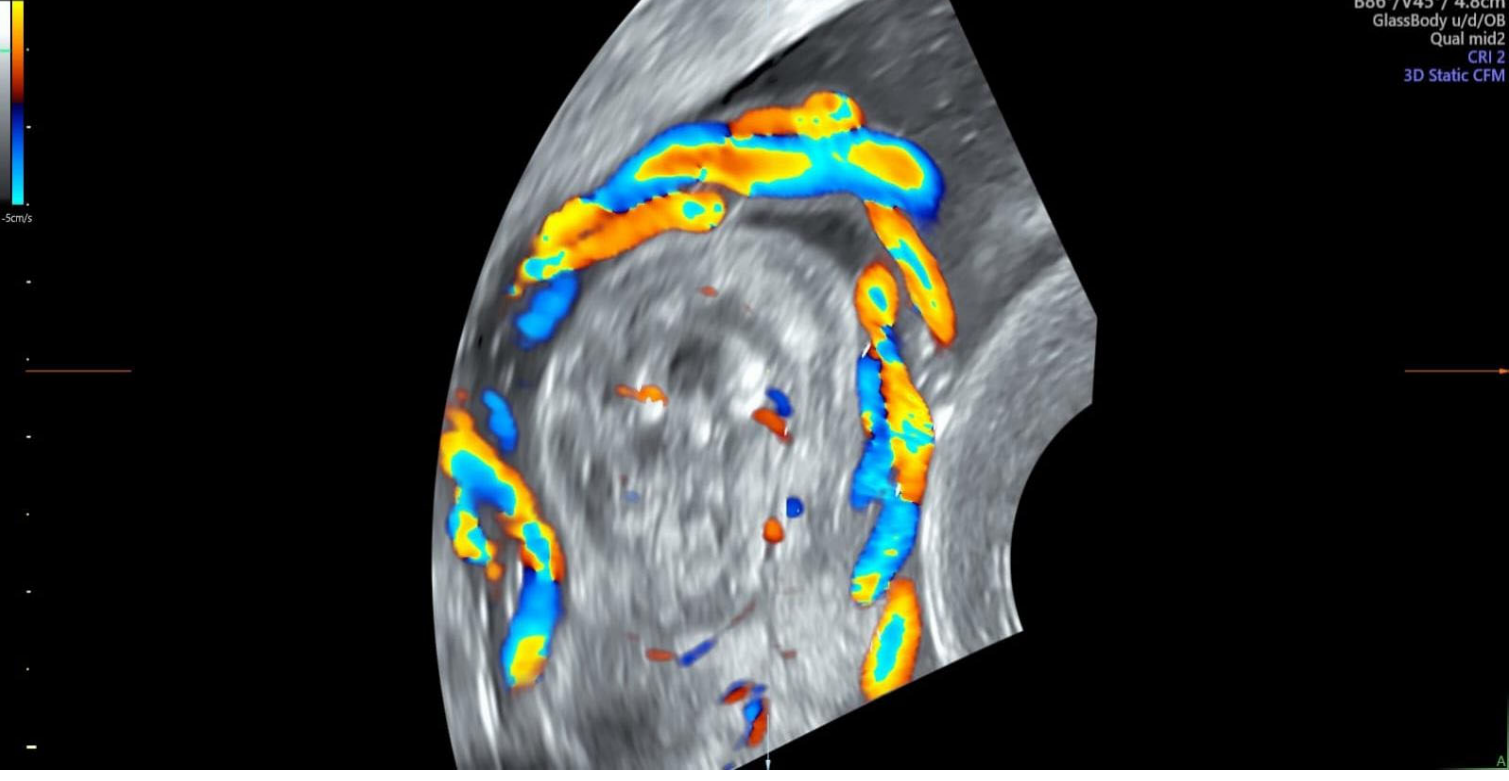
Ultrasound entanglement from right to left of umbilical cord around the fetal neck. Blood flow characteristics: the right half ring has two blue and one red flows, while the left half ring has two red and one blue flows, which shows that the blood flow is anticlockwise and suggests that the umbilical cord on the neck coils from left to right.
